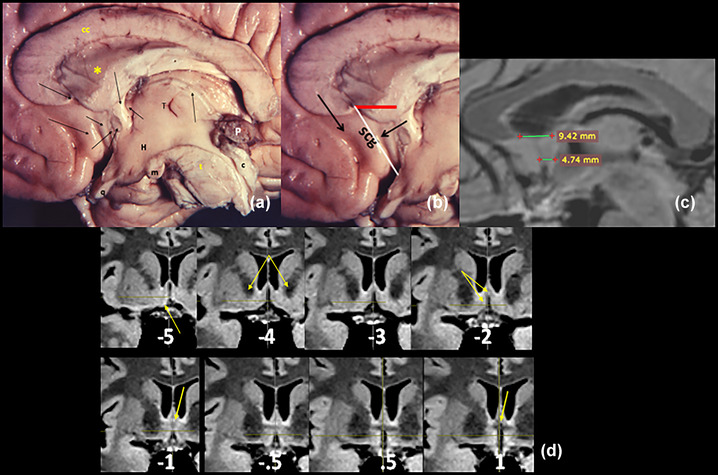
Medial structures intimately related to the anterior perforated substance (APS). (a) cc, corpus callosum; star, septum lucidum with anterior septal vein; first horizontal row of black arrows, left to right—beak of the corpus callosum, poscommissural fornix, foramen of Monro, stria medullaris; second row—subcallosal gyrus (scg); paraterminal gyrus or vertical limb of Broca's band (vdbB); lamina terminalis; anterior commissure (ac). H, hypothalamus; q, optic chiasm; m, mammillary body; t, mesencephalic tegmentum; c, collicular plate; P, pineal gland. (b) Anterior and posterior paraterminal sulci— white line, anterior limit of the septum. (c) MRI image from our model measured with Radiant DICOM Viewer. (d) Coronal cuts are better for analyzing the septum. In our model, a run of slices perpendicular to the CA–CP plane from 5 mm ahead of the ca, till 1 mm behind it. In the last slice (right below), the triangular recess of the III V (yellow arrow); in −0.5 and −1, crossing of the vdbB in front of the ca; in −5, beginning of the scg (yellow arrow). Ahead of there, the fissure becomes progressively closed by the thick scg. In −2 yellow arrows, possible Ch1 and Ch 2 nuclei. Ch 1 seems to prolong itself till −0.5. In all the slices in the inferior row, the presence of the hypothalamus‐preoptic region makes the septum to be only pre‐ and supracommissural.
